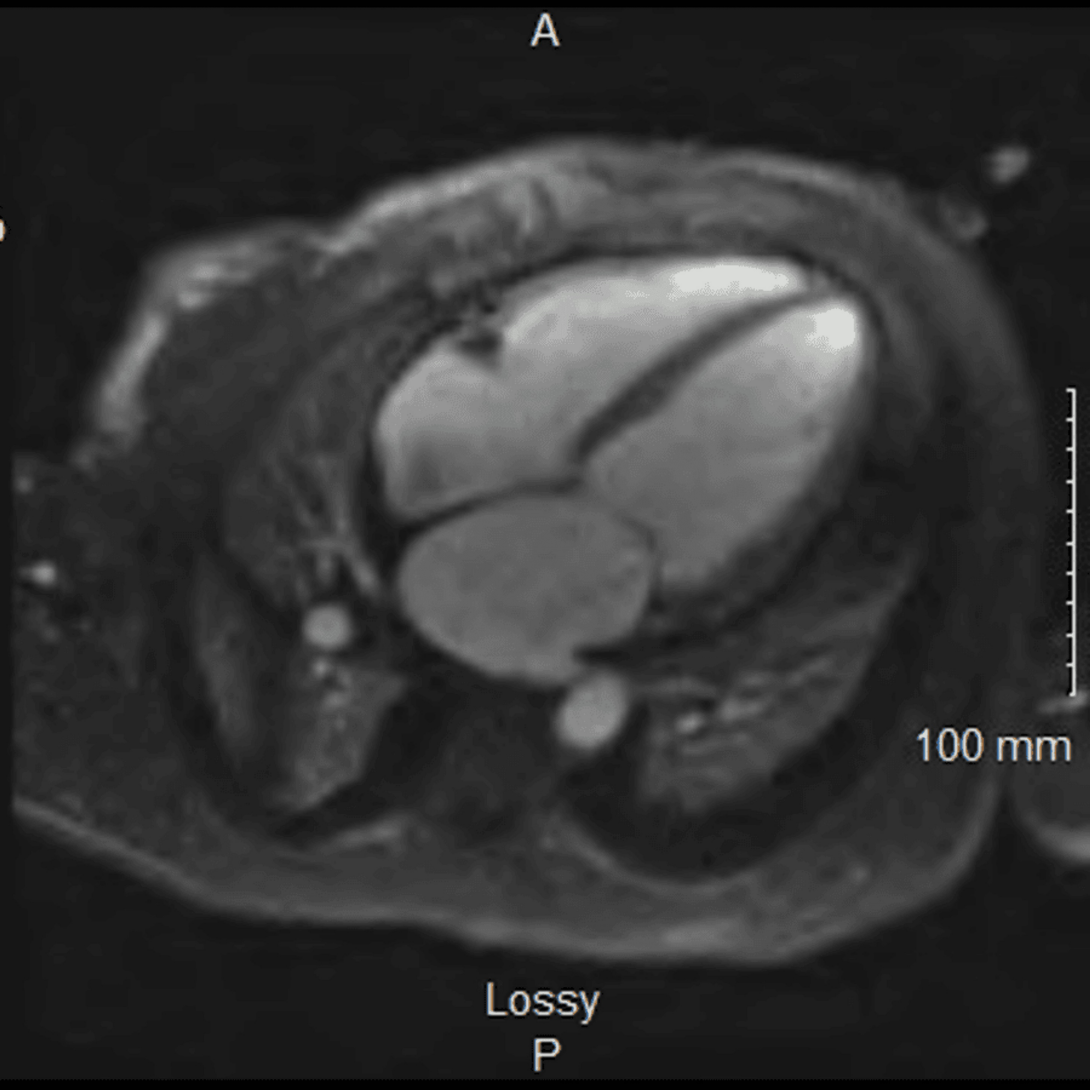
Cardiac MRI with globally diminished left ventricular systolic function, with global left ventricular hypokinesis, left ventricular dilatation, and no definitive delayed myocardial enhancement to suggest inflammatory or infectious myocarditis.
